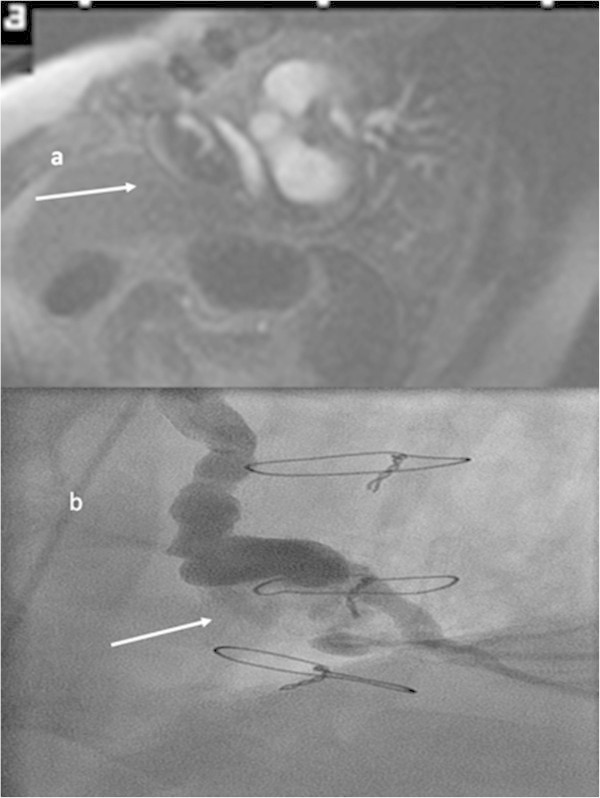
Aneurysm in MRI and coronary angiography. a: Perfusion of the aorta and the bypass aneurysm is seen at the same time (white arrows, parasagittal views, application of contrast medium, TR: 174, TE: 0.94). b: Coronary angiography (LAO 30 0) revealed a large aneurysm of the degenerated venous graft of the right coronary artery (RCA) with a thrombus and small leakage into the ventricle (white arrow). The distal part of the native RCA (occluded at the ostium) is promptly perfused with the graft.
